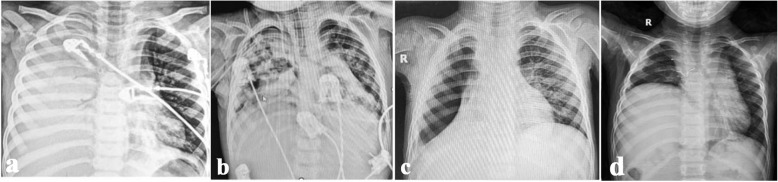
3-year-old child presented with a) severe respiratory distress, pancytopenia, rapidly progressive consolidation and highly septated effusion. Respiratory support escalated quickly form high flow nasal cannula to high frequency oscillatory ventilation in 12 h. Two days later, laboratory picture changed to leukocytosis, severe anemia and extreme thrombocytosis. Fibrinolytics were not administered as the patient was hemodynamically unstable. Nasopharyngeal respiratory panel by multiplex PCR was positive for adenovirus, *H influenza and S. pneumoniae.* Pleural fluid and endotracheal aspirate cultures showed no growth of organisms. Blood culture was positive for Staphylococcus Hominis. After 60 days of hospital admission, he had bronchopleural fistula, persistent air leak and lung collapse intractable to drainage and suction (b, c). He was referred for surgery 3 months after discharge. Decortication and right lower lobectomy were performed. Follow up chest X-ray 2 months after surgery d) shows compensatory hyperinflation on the contralateral side
